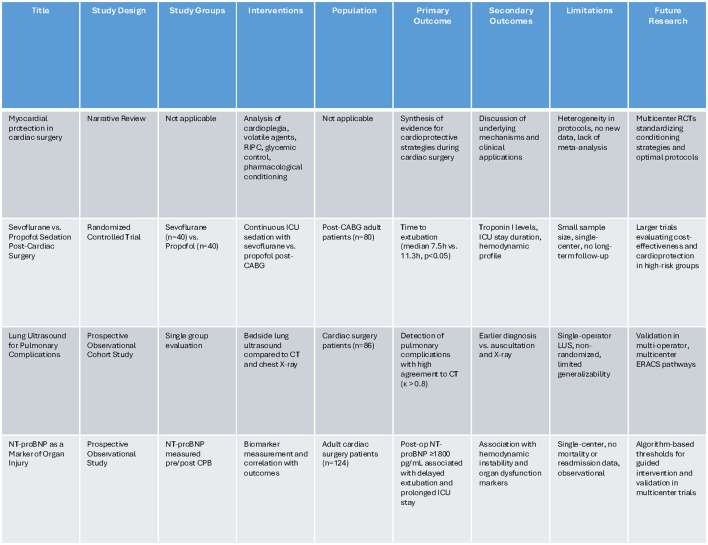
A table compares four studies on cardiac surgery advancements. Columns include Title, Study Design, Study Groups, Interventions, Population, Primary Outcome, Secondary Outcomes, Limitations, and Future Research. Rows cover topics like myocardial protection, sedation techniques, lung ultrasound for complications, and NT-proBNP as a marker of organ injury. Each study is detailed with specific methodologies, outcomes, and suggestions for future research including larger trials and validation efforts. Limitations include small sample sizes and generalizability issues.
